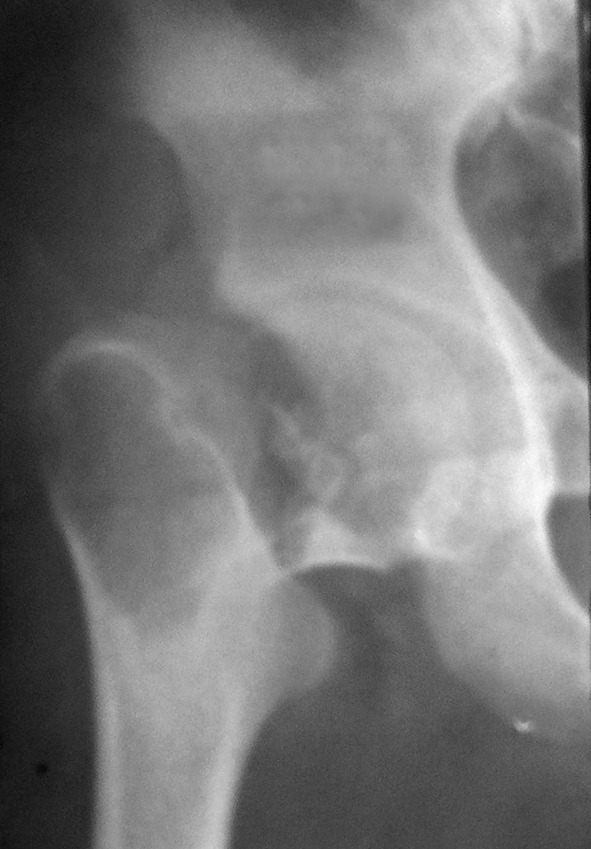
Antero-posterior radiograph showing an osteolytic lesion in the proximal femur with a pathological femoral neck fracture
